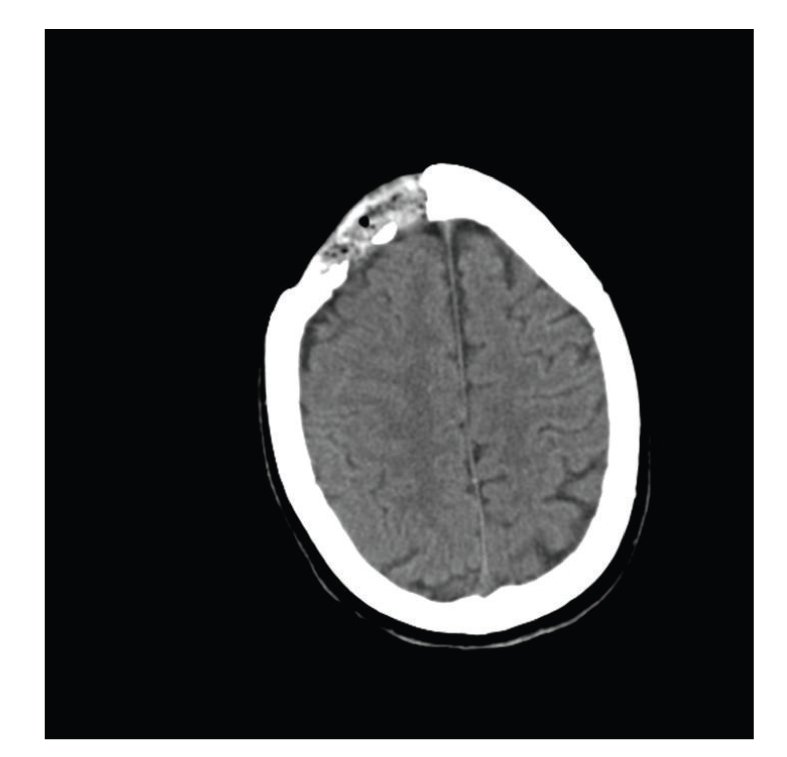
Head CT showing encephalomalacia in the right frontal lobe.
